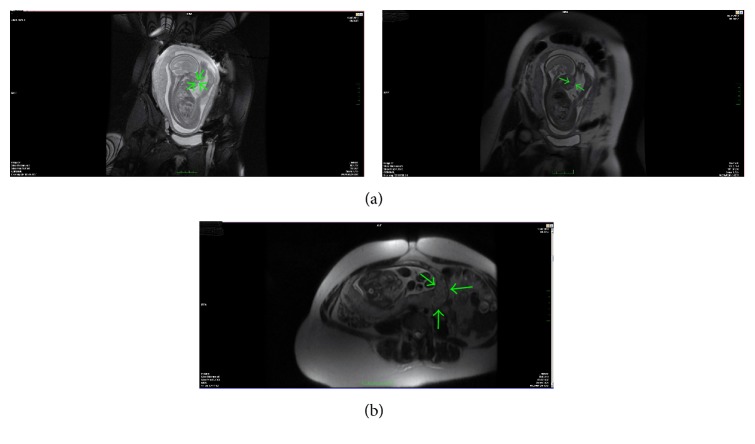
The MRI images of the fetus after first diagnosis of epignathus, green arrows show the mass.
